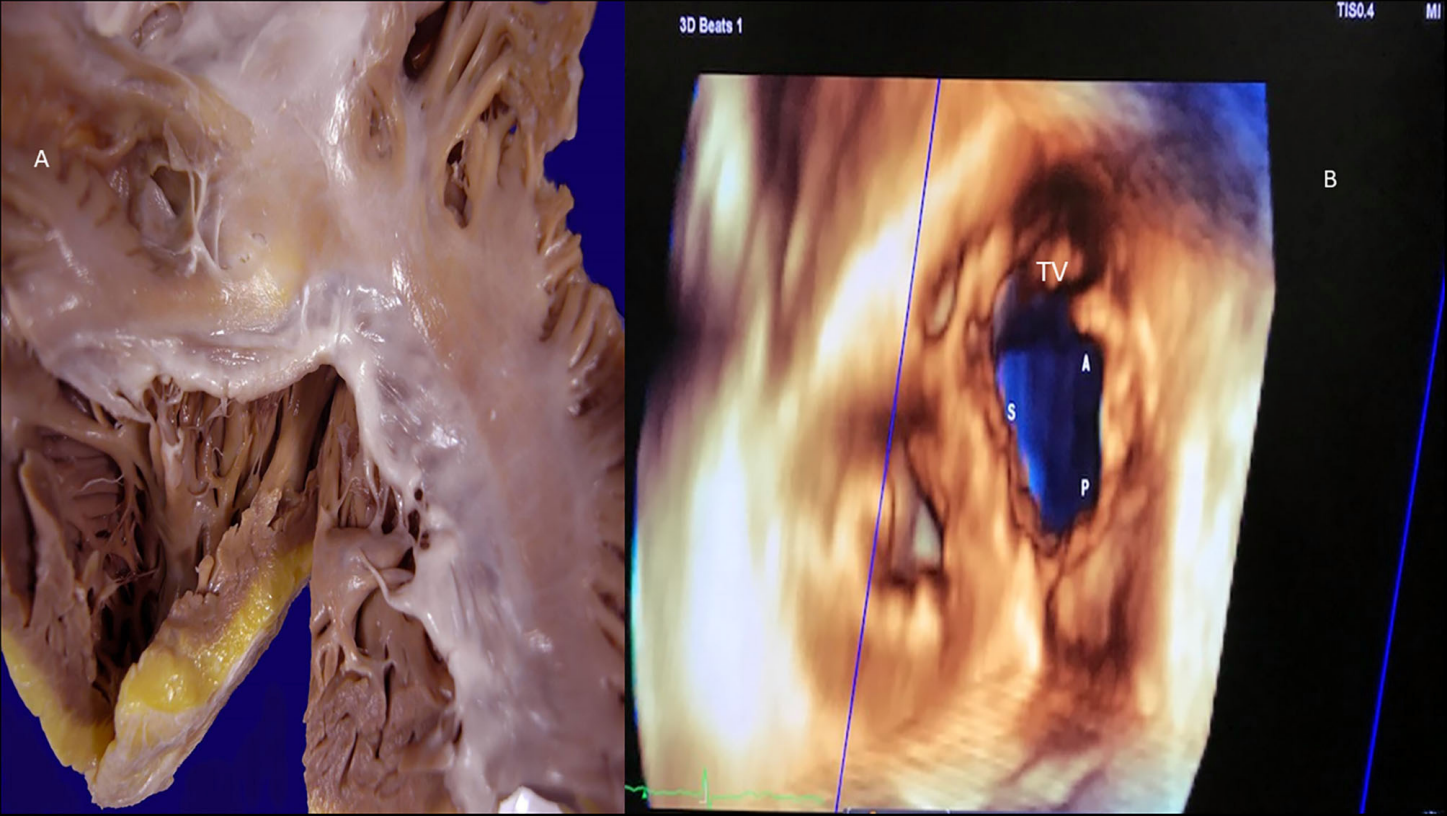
Tricuspid valve rheumatic analysis. (A) Macroscopy: the tendinous cords are thickened, fused, and retracted (lack of intercordal spaces), and the anteroseptal commissure is fused. The leaflets show thickening of the free edges. (B) 3D echocardiography (right atrial view). Demonstration of lack of coaptation leading to an important regurgitation. S, septal cusp; A, anterior cusp; P, posterior cusp.
